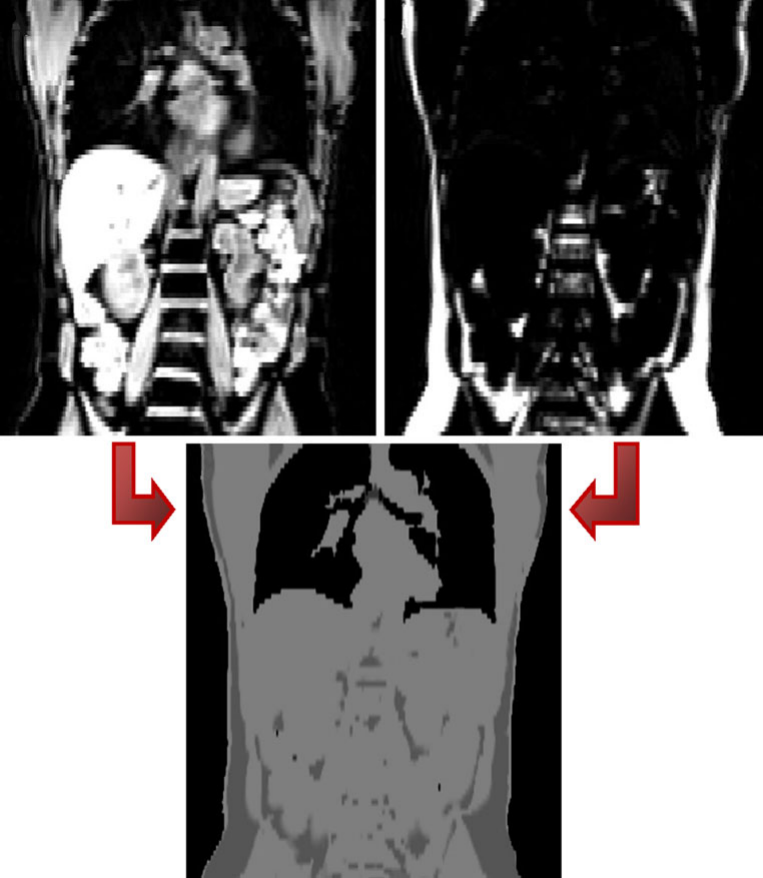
Dixon-based segmentation for whole-body attenuation correction shown in [44] (courtesy of A. Martinez-Moeller): MRI water (*top left*) and fat (*top right*) images acquired with a 2-point Dixon sequence are combined and segmented to generate the attenuation map for lungs, adipose tissue, soft tissue, and background
